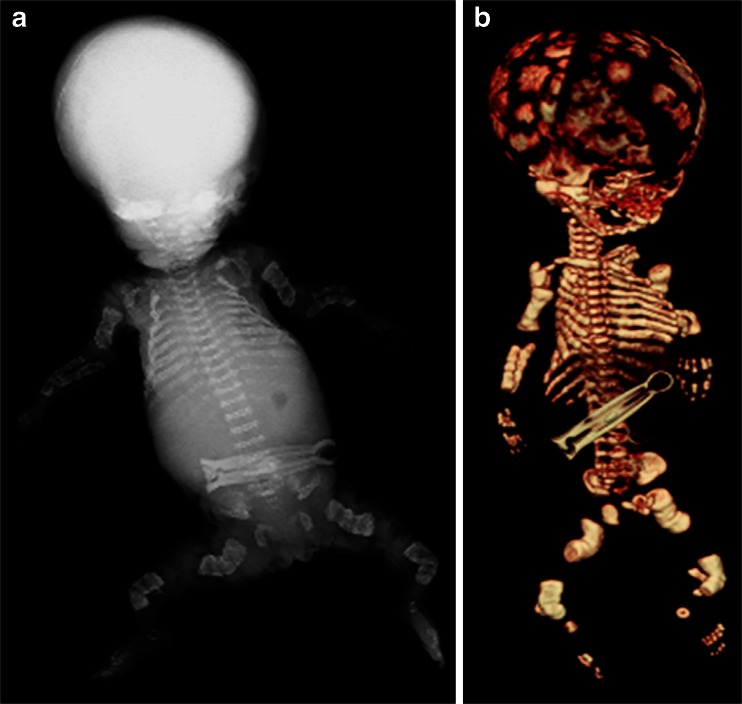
Postmortem imaging in a male fetus of 20 weeks gestation suspected of having skeletal dysplasia. Both postmortem radiograph (a) and postmortem CT image (b) of a foetus terminated for suspected skeletal dysplasia show crumpled long bones and ribs, representing multiple fractures of osteogenesis imperfecta type II. Image (a) reproduced with permission [16]
